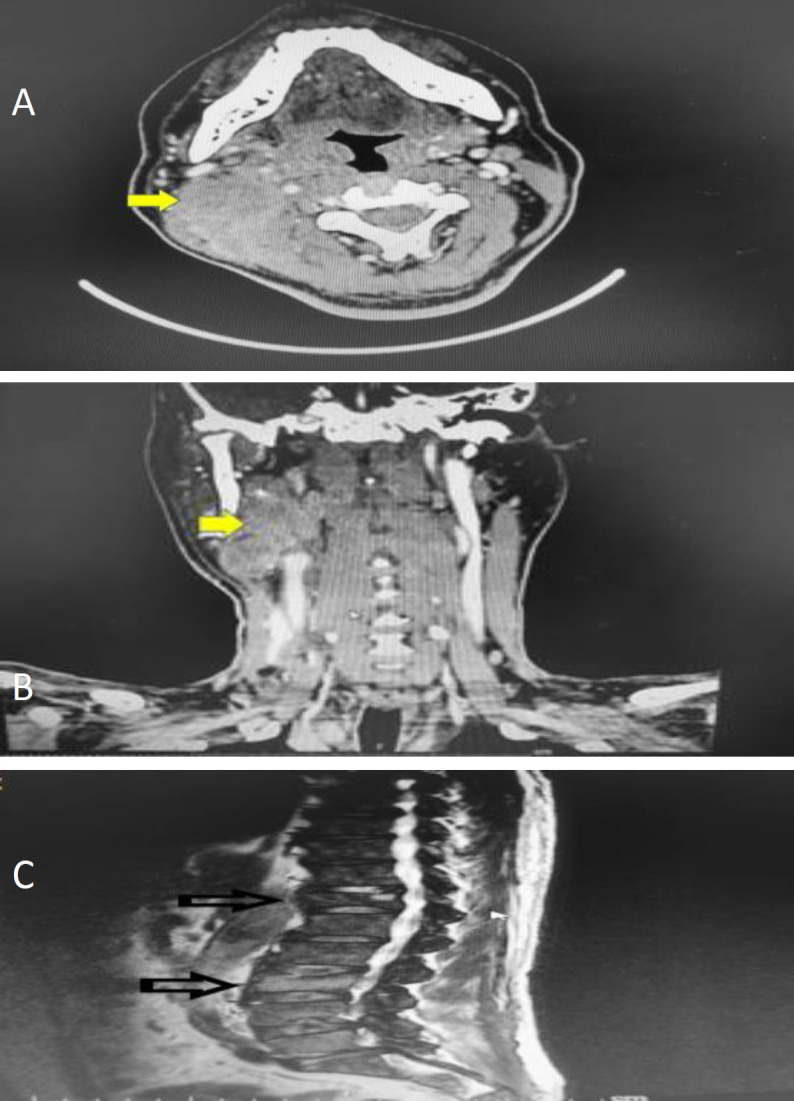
A. CT Neck (axial view) showed lesion at right tonsil extend to parapharyngeal region (yellow arrow), B. CT Neck (coronal view) showed lesion at right parapharyngeal region (yellow arrow), C. T2 sagittal view of the MRI of the thoracolumbar vertebrae showed pathological compression fracture at the level of T12 and L3 (black arrow)
